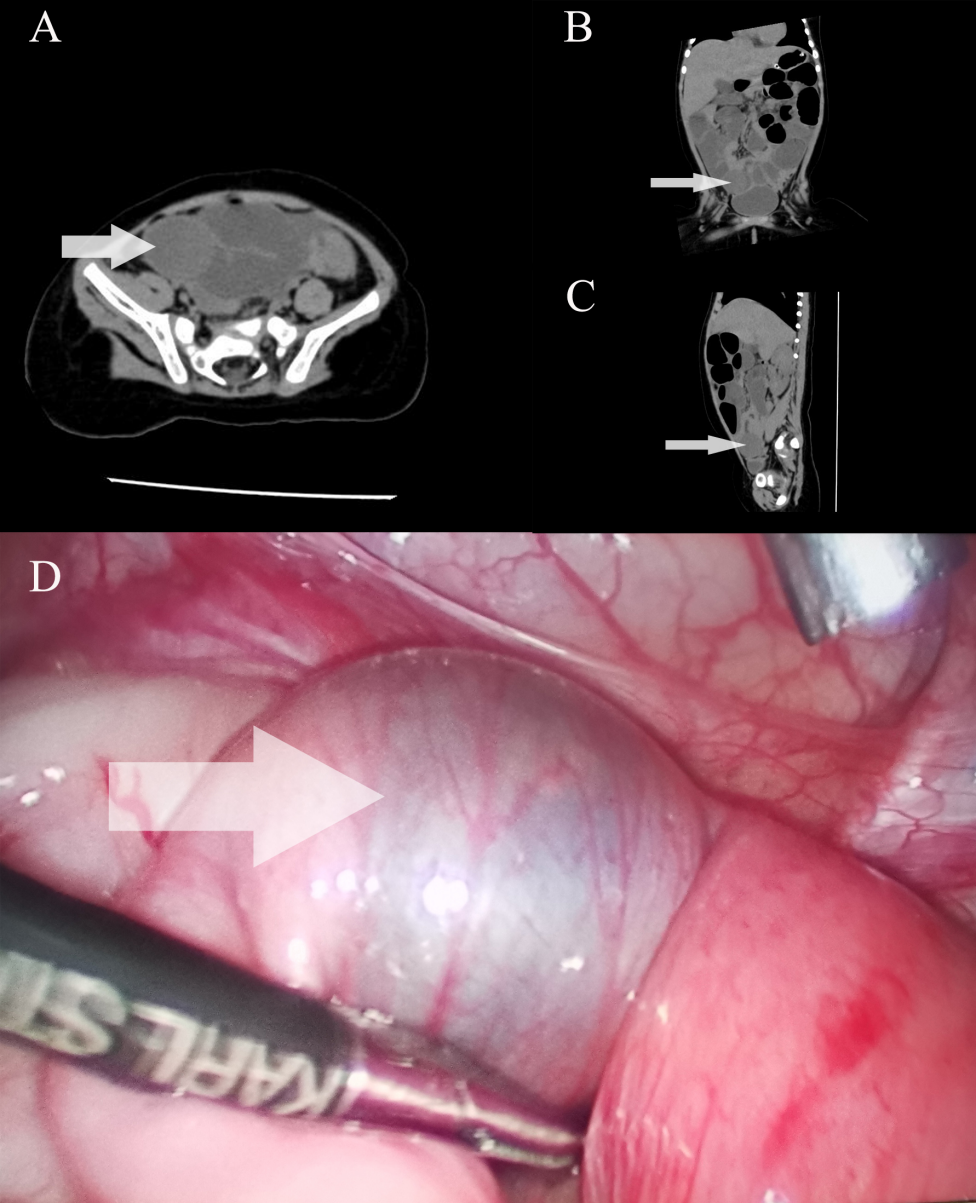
Imaging and intraoperative findings of Shine-Muscat grape-induced small bowel obstruction in an infant. Abdominal CT scans in axial (A), coronal (B), and sagittal (C) planes, clearly showing the location of the foreign body (Shine-Muscat grape) causing small bowel obstruction. The arrows indicate the exact position of the grape in the intestinal lumen, which appears as a well-defined round mass causing luminal narrowing and obstruction. (D) Intraoperative view of the foreign body located within the terminal ileum. The image shows a visibly distended proximal bowel loop (marked by the arrow), while the distal bowel loops appear collapsed. The obstruction was relieved after the surgical removal of the Shine-Muscat grape, which was intact and causing a mechanical blockage.
